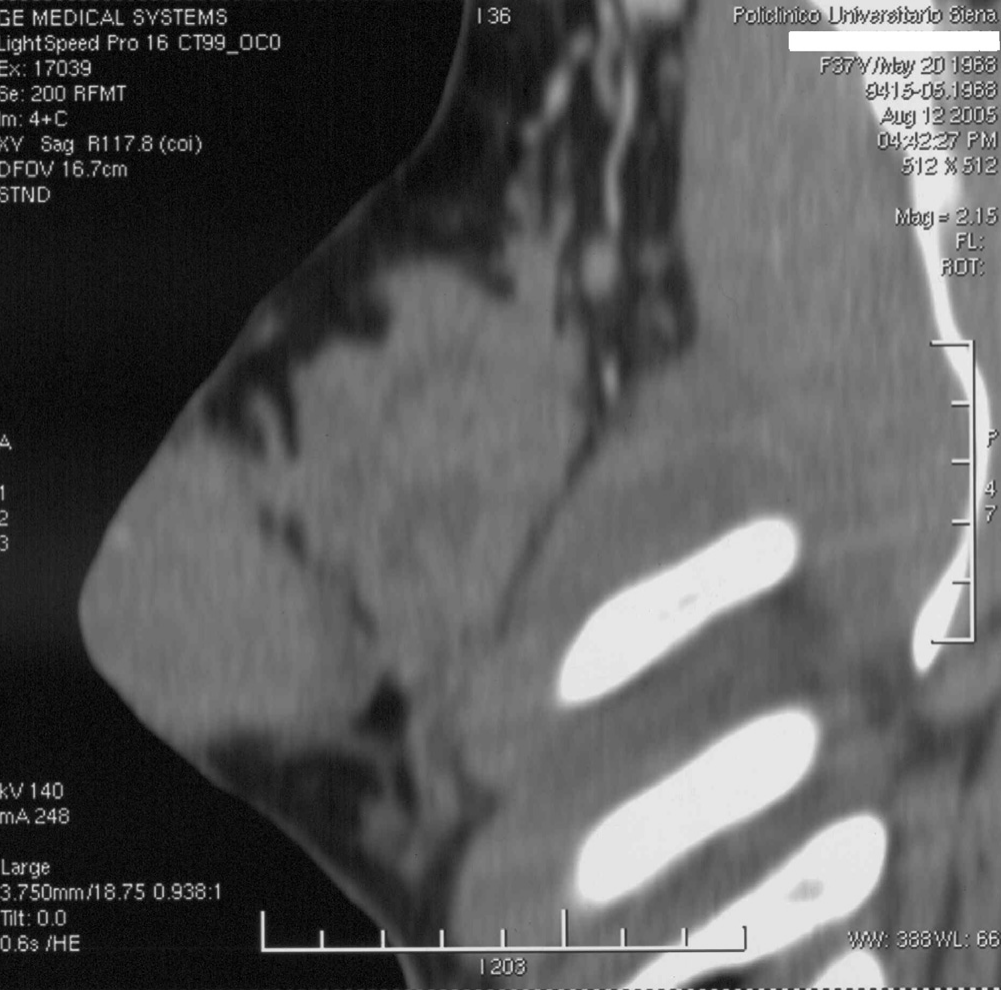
A CT scan shows a large breast mass associated with pathological enlarged lymph nodes in the right axilla.
